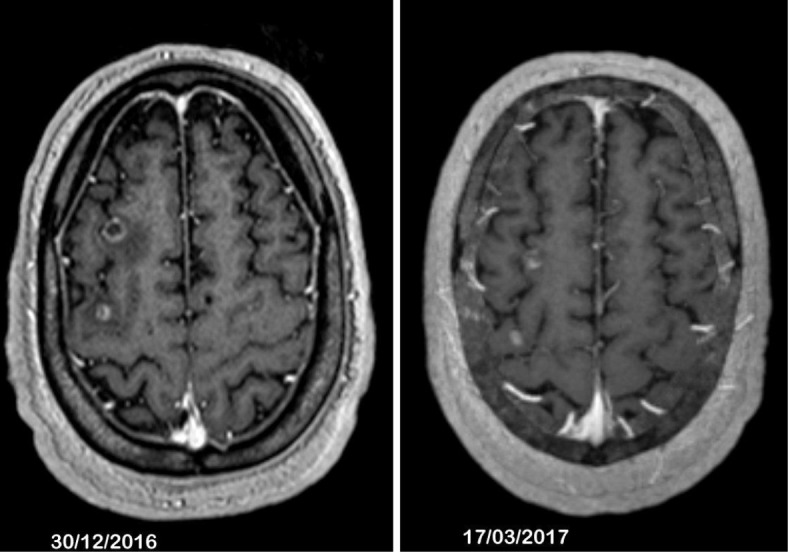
Brain magnetic resonance imaging of Case 2, effect of cabozantinib after 2.5 months
